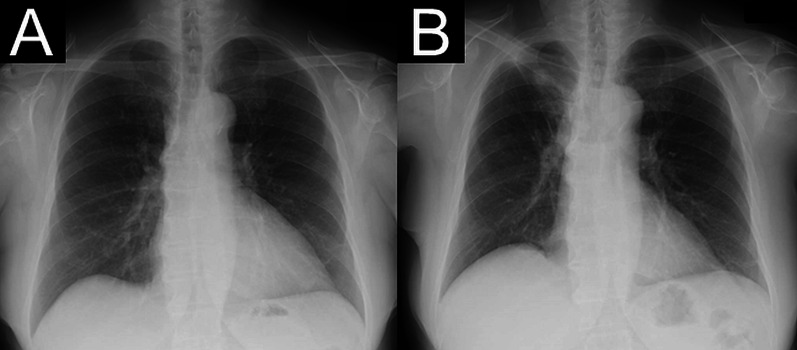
Chest X-ray before and after surgery. A Chest X-ray taken before surgery. B Chest X-ray taken 1 month after surgery
